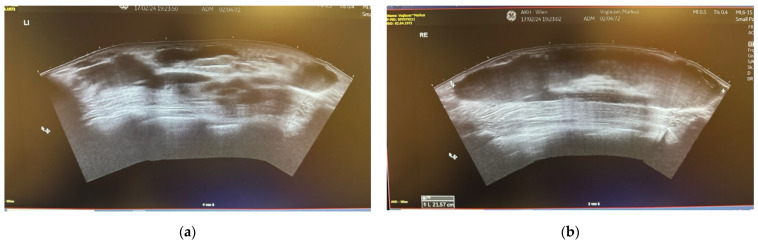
(a) Ultrasound of the left breast region; (b) Ultrasound of the right breast region.
